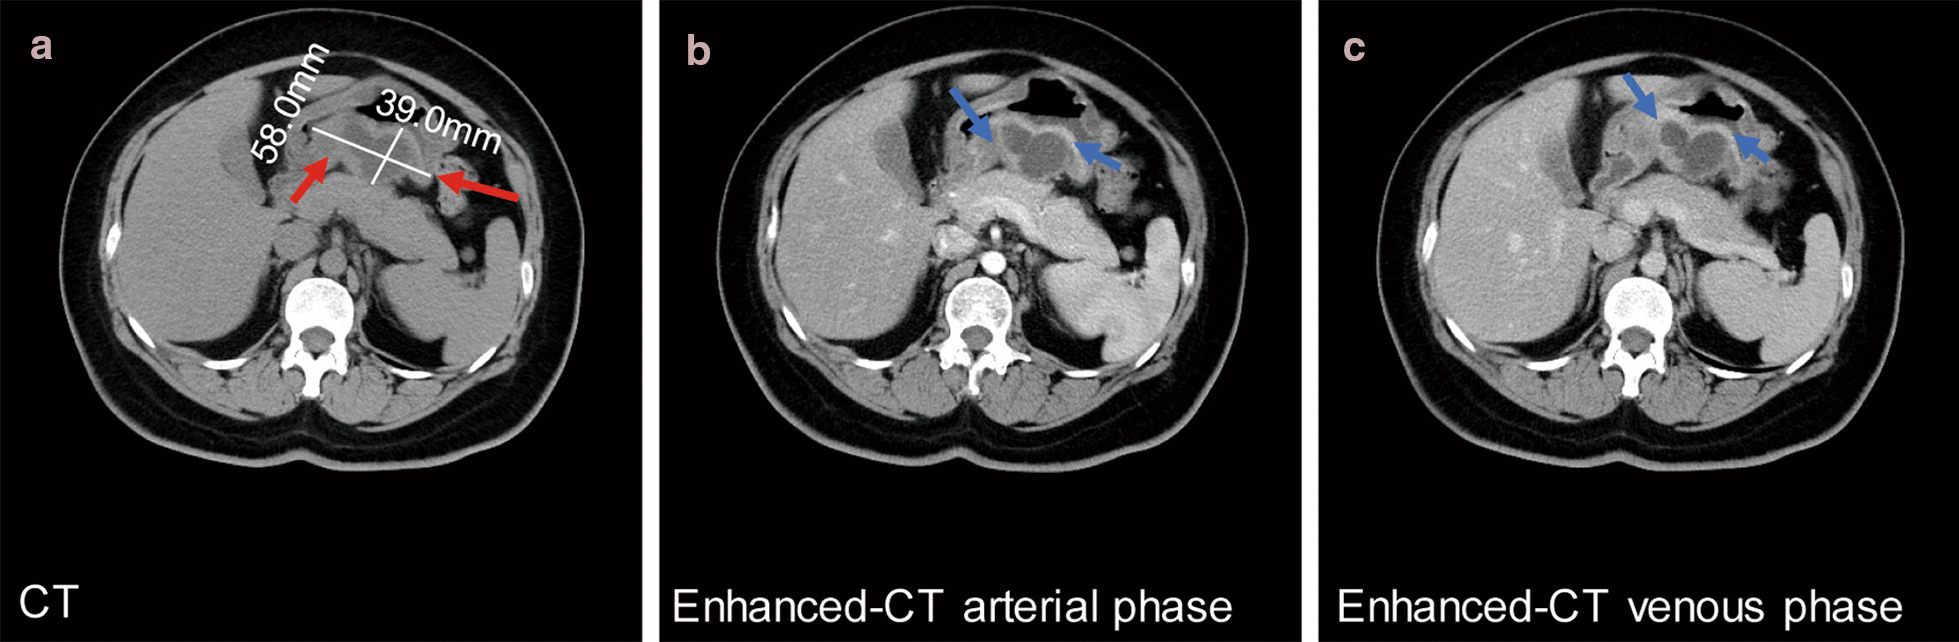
A heterogeneous cystic and solid submucosal tumor in the stomach was detected by CT. a The size and location of the tumor are shown on CT by the red arrows. b, c The enhanced CT scans in the arterial phase and venous phase, respectively, and blue arrows indicate thick cystic walls
